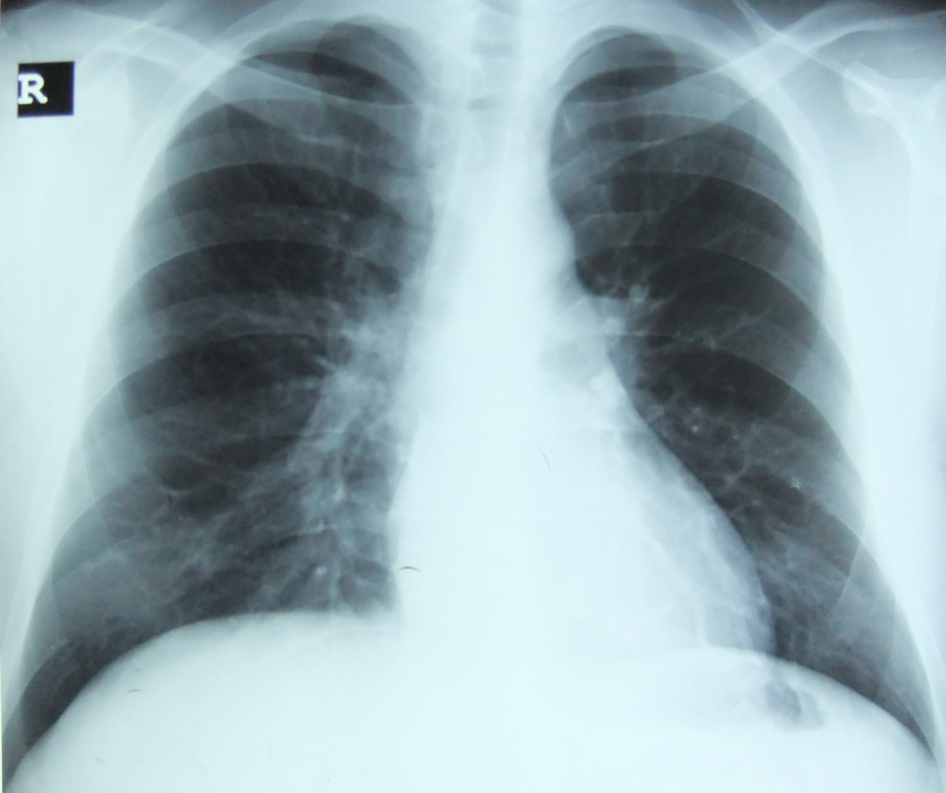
Chest radiography shows bronchovascular prominance on admission.
